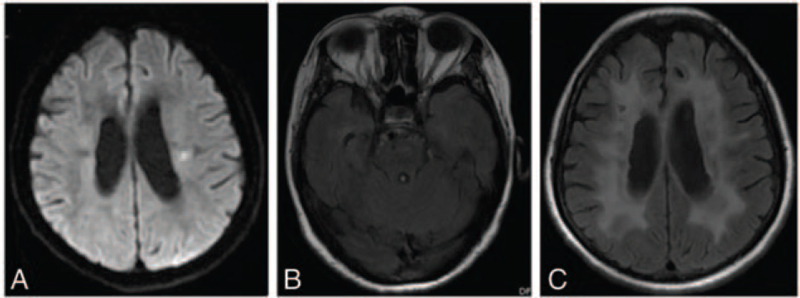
MRI images of the brain of the patient. (A) The diffusion-weighted image (DWI) shows acute lacunar subcortical infarction. (B) The axial T2-weighted image shows no white matter change in temporal lobes. (C) The fluid attenuated inversion recovery (FLAIR) shows extensive confluent white matter lesions at periventricular region.
